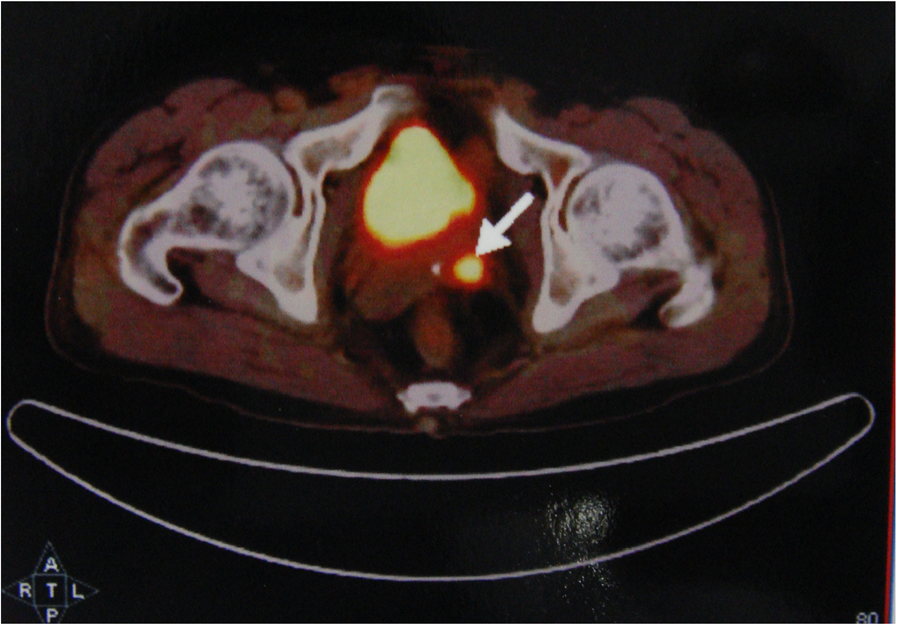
Positron emission tomography/computed tomography (PET/CT). There was still tumor activity in the left seminal vesicle.
